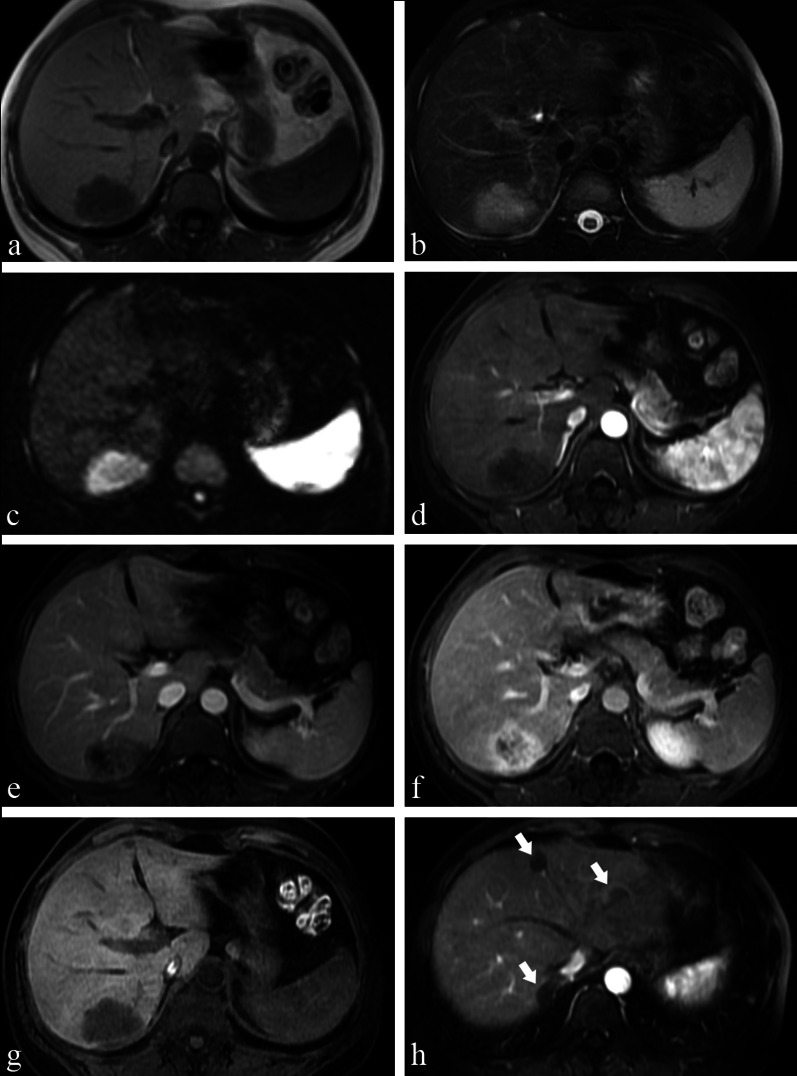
A 36-year-old female with pathologically diagnosed HEHE. Axial unenhanced T1-weighted MRI (a) demonstrates a hypointense tumour in segment 6 of the liver, which is hyperintense on axial fat-suppressed T2WI (b) and DWI (c). On contrast-enhanced images of three phases (d, f), the tumour presented enhancement pattern A, with peripheral irregular enhancement on arterial phase (d), with centripetal progressive filling on the portal venous phase and (e) equilibrium phase (f) along with gradually increasing intensification, and a “lollipop sign” can be seen on portal venous phase. The tumour manifested as a homogeneous hypo-intensity on the HBP (g). There were other lesions (arrows) in the upper part of the liver on the arterial phase (h) with pathologically proven epithelioid haemangioendothelioma
